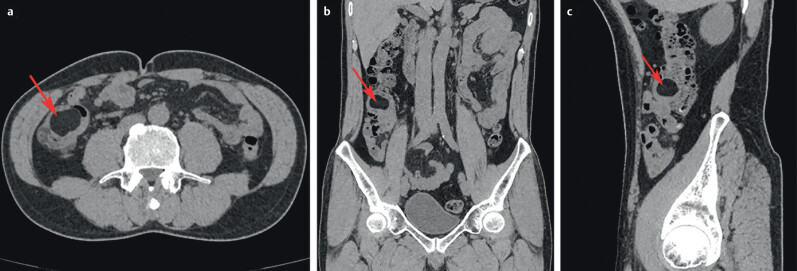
Computed tomography revealed a benign space-occupying lesion
